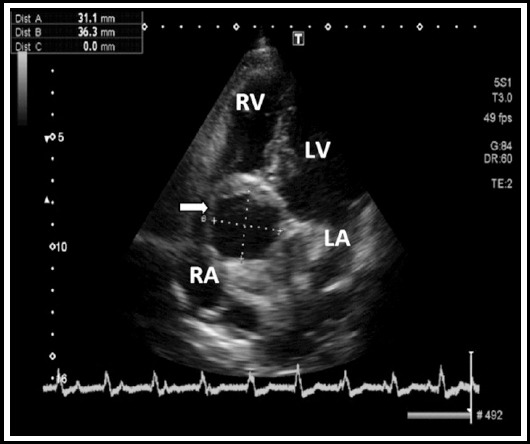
Transthoracic echo apical 5 chamber view showing non-coronary sinus aortic aneurysm (RV = Right Ventricle, LV = Left Ventricle, RA = Right Atrium, LA = Left Atrium).
